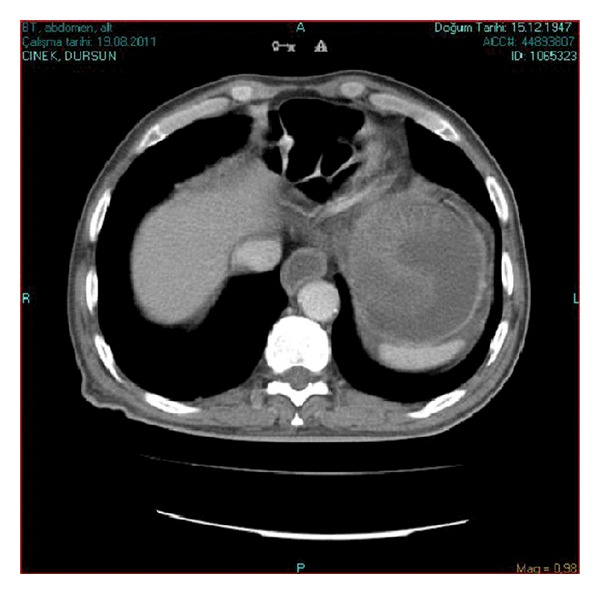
Emergency CT scan of the abdomen. Dilated stomach with intragastric nonhomogeneous mass compatible with bowel loops.
